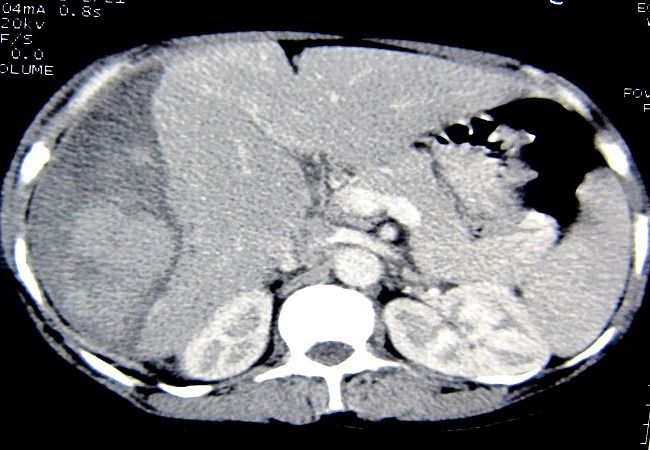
Axial CT scan showing large liver hematoma compressing the liver
